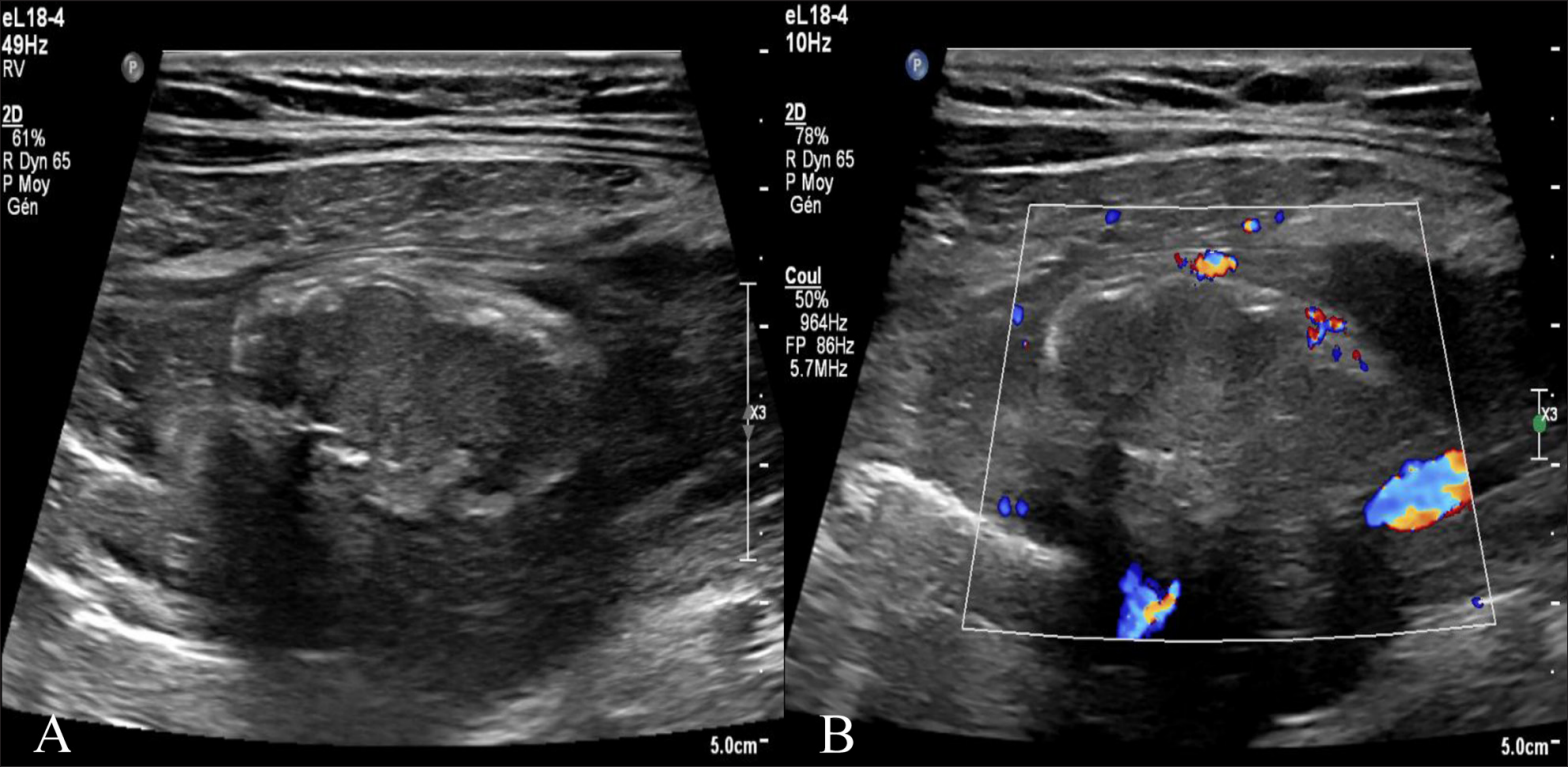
Right iliac fossa ultrasound. A) Superficial intraperitoneal oblong mass in the right iliac fossa of 35mm non-compressible, hyperechoic, surrounded by a subtle hypoechoic line. B) without internal vascularity.
